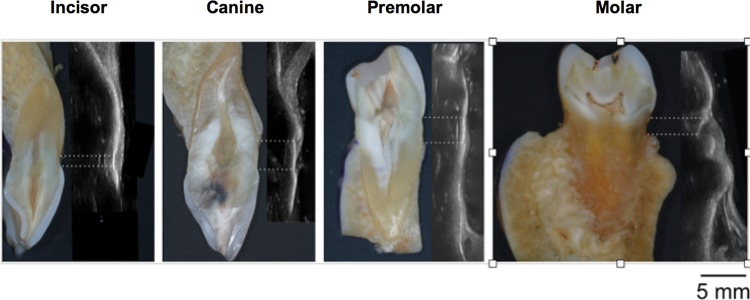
Demonstrations of ultrasound images for different tooth types in relation to the ground sections of the respective teeth. The two dashed lines represent the CEJ and alveolar bone crest level, respectively.
